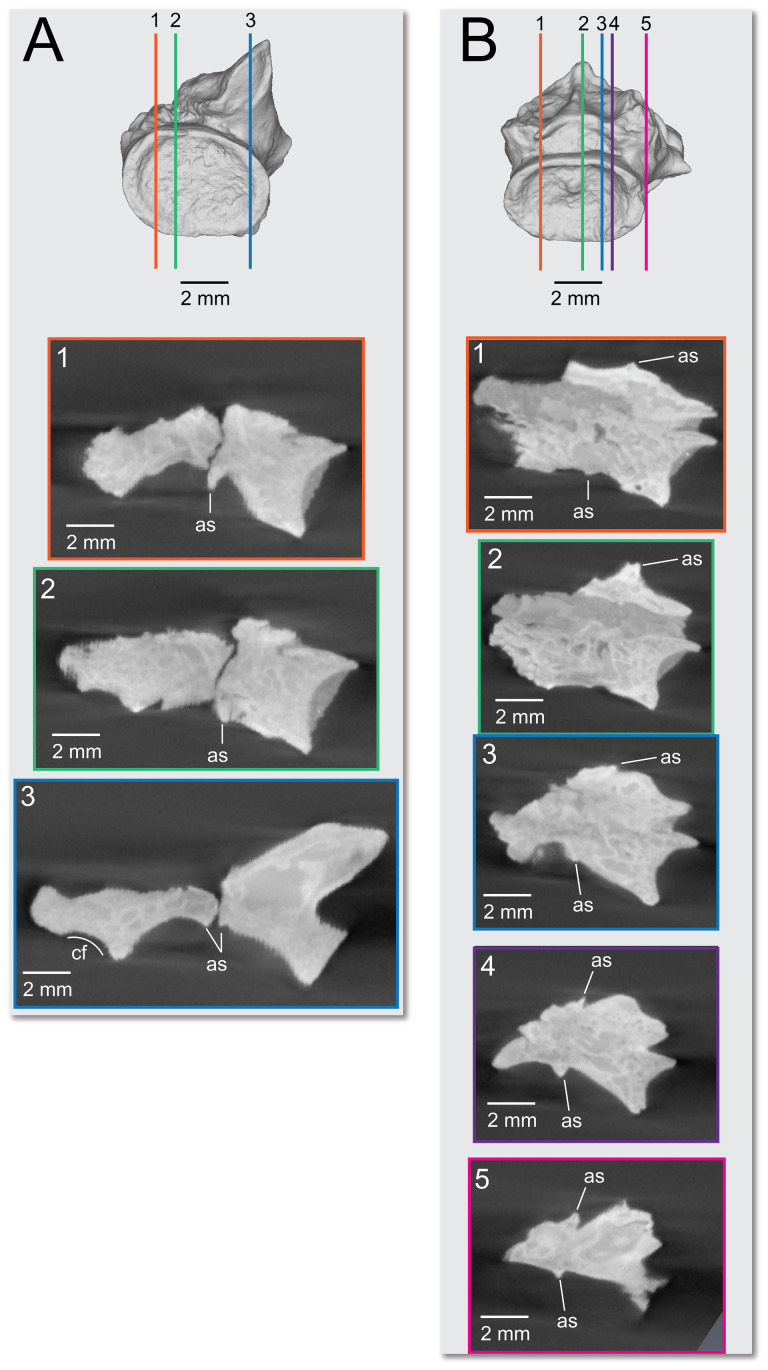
Sagittal CT slices of autotomy septa in (A) distal caudal vertebra UMNH VP 16266.29 and (B) distal caudal vertebra UMNH VP 16266.30. Location of slices indicated by coloured vertical lines in (A) and (B). Anatomical abbreviations: as, autotomy septum; cf, chevron facet.
